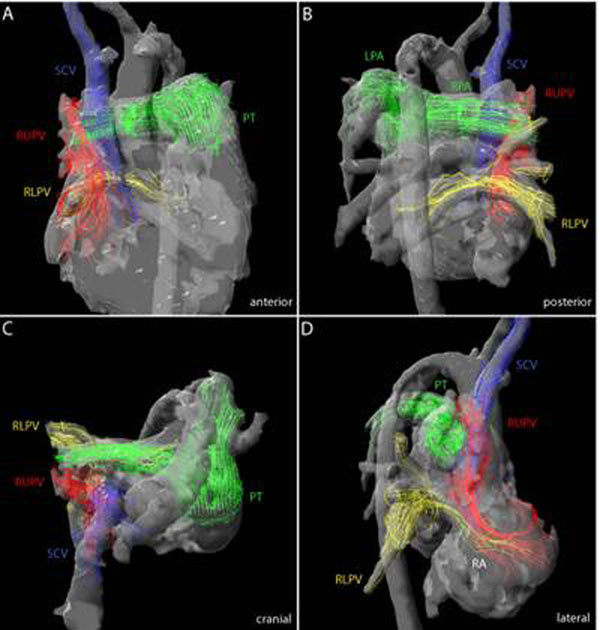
# Selective pulmonary venous flow visualization and quantification by flow-sensitive four-dimensional cine magnetic resonance imaging facilitates and improves the accurate diagnosis of partial anomalous pulmonary venous drainage
